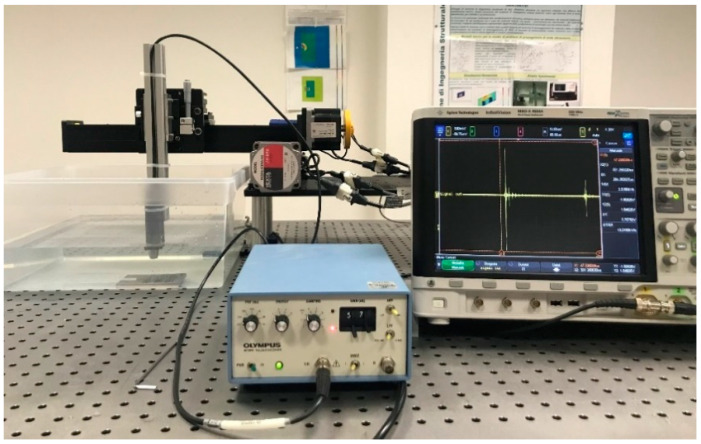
Ultrasonic immersion tests setup: main components.
